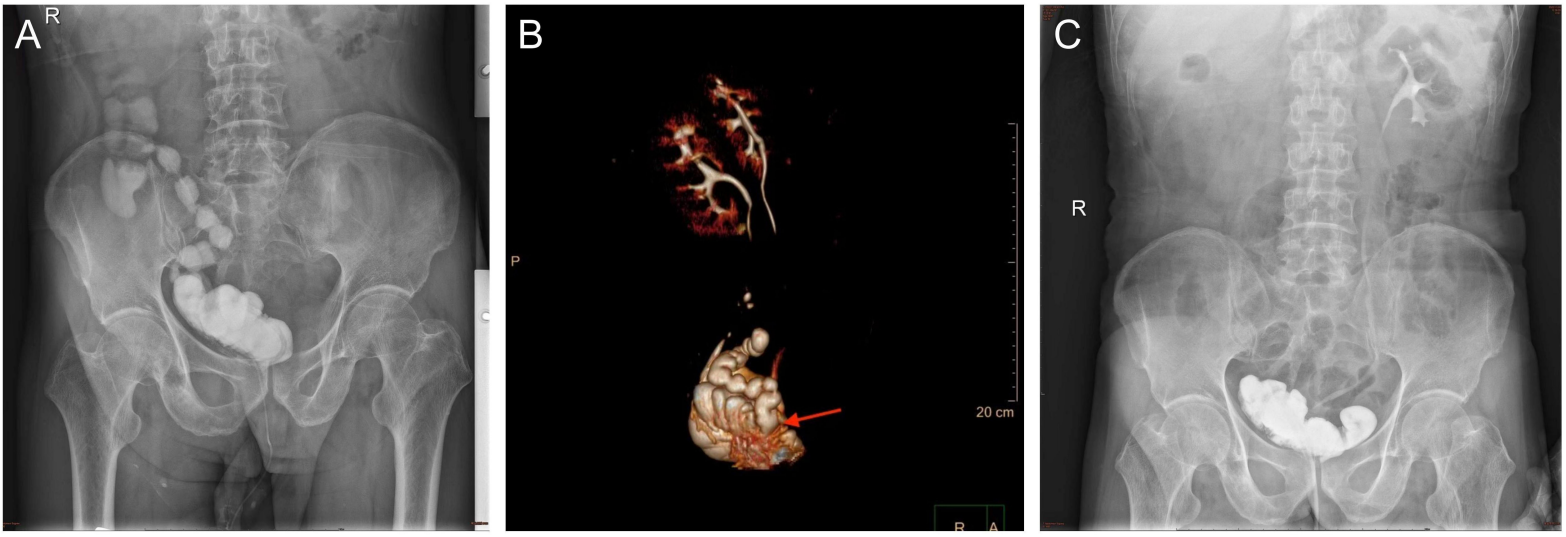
(A) The image of cystography examination showed the contrast agent was found in the ileum and right colon before the surgery. (B) Computed tomography urography reconstructed the spatial position of the neobladder and ileum. (C) The image of cystography examination showed the contrast agent was not found in the ileum and right colon after the surgery.
